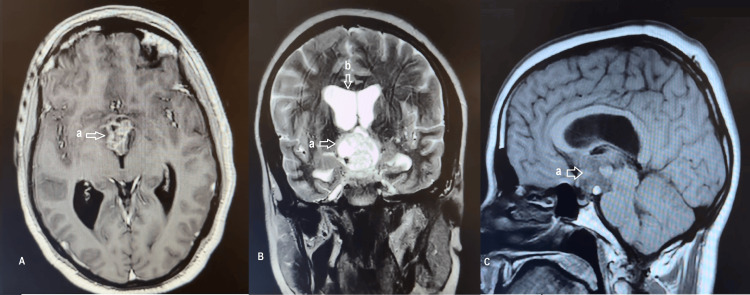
Brain MRI of case 2 showing a suprasellar solid-cystic lesion measuring 32×27×27 mm, causing mild biventricular hydrocephalus and exhibiting hemorrhagic changes. a: suprasellar solid-cystic lesion measuring 32×27×27 mm. b: biventricular hydrocephalus. A: Preoperative axial T1-weighted brain MRI sequences showing a suprasellar lesion. B: Preoperative axial T2-weighted brain MRI sequences showing a suprasellar solid-cystic lesion measuring 32×27×27 mm, causing mild biventricular hydrocephalus and exhibiting hemorrhagic changes. C: Preoperative sagittal T1-weighted brain MRI sequences showing a suprasellar solid-cystic lesion exhibiting hemorrhagic changes.
